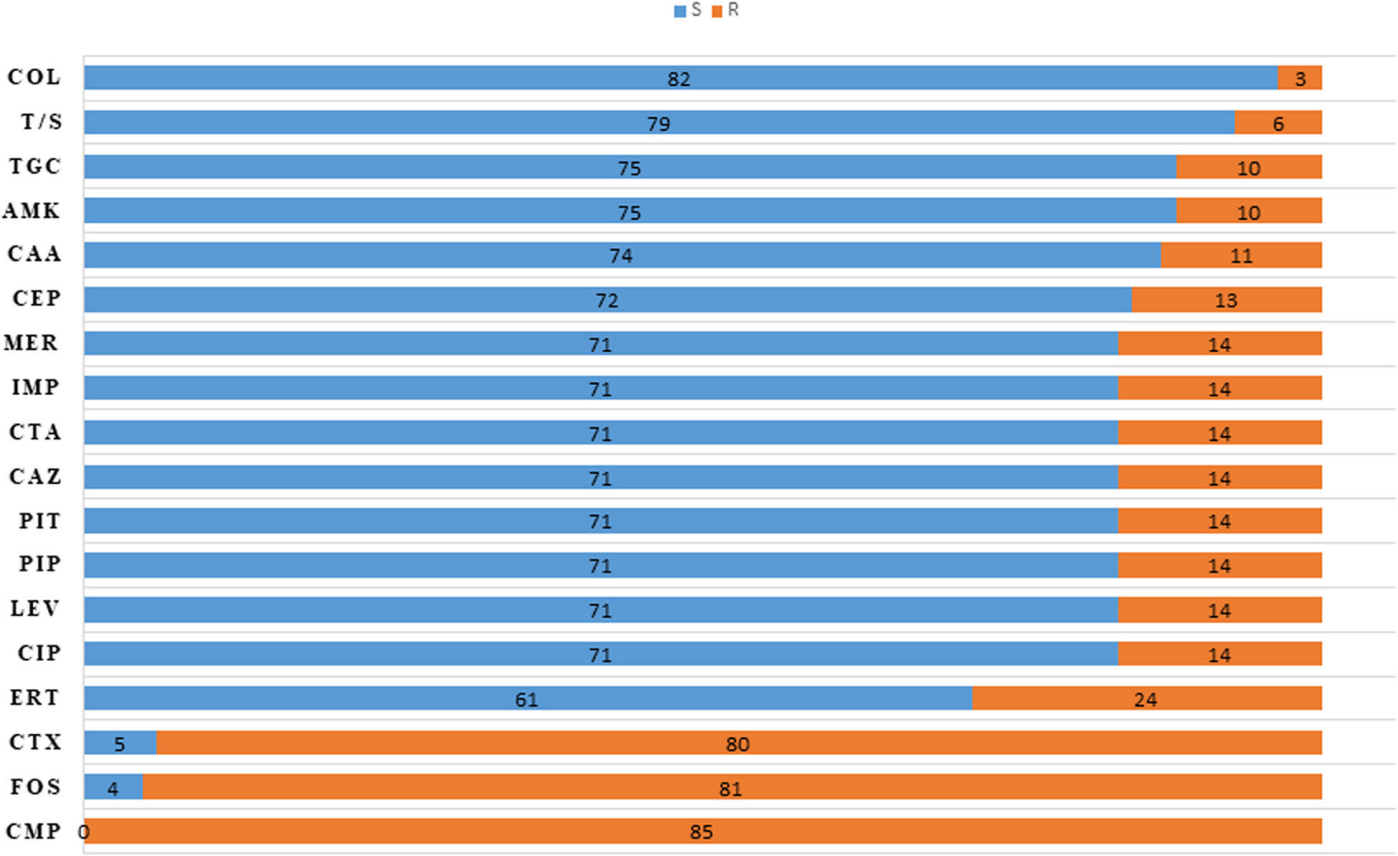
Number of resistant and sensitive isolates among 85 *A. baumannii* strains isolated from human and milk powder samples in Germany. COL, Colistin; T/S, Trimethoprim/Sulfamethoxazole; TGC, Tigecycline; AMK, Amikacin; CAA, Ceftazidime/Avibactam; CEP, Cefepime; MER, Meropenem; IMP, Imipenem; CTA, Ceftolozane/Tazobactam; CAZ, Ceftazidime; PIT, Piperacillin/Tazobactam; PIP, Piperacillin; LEV, Levofloxacin; CIP, Ciprofloxacin; ERT, Ertapenem; CTX, Cefotaxime; FOS, Fosfomycin; CMP, Chloramphenicol
